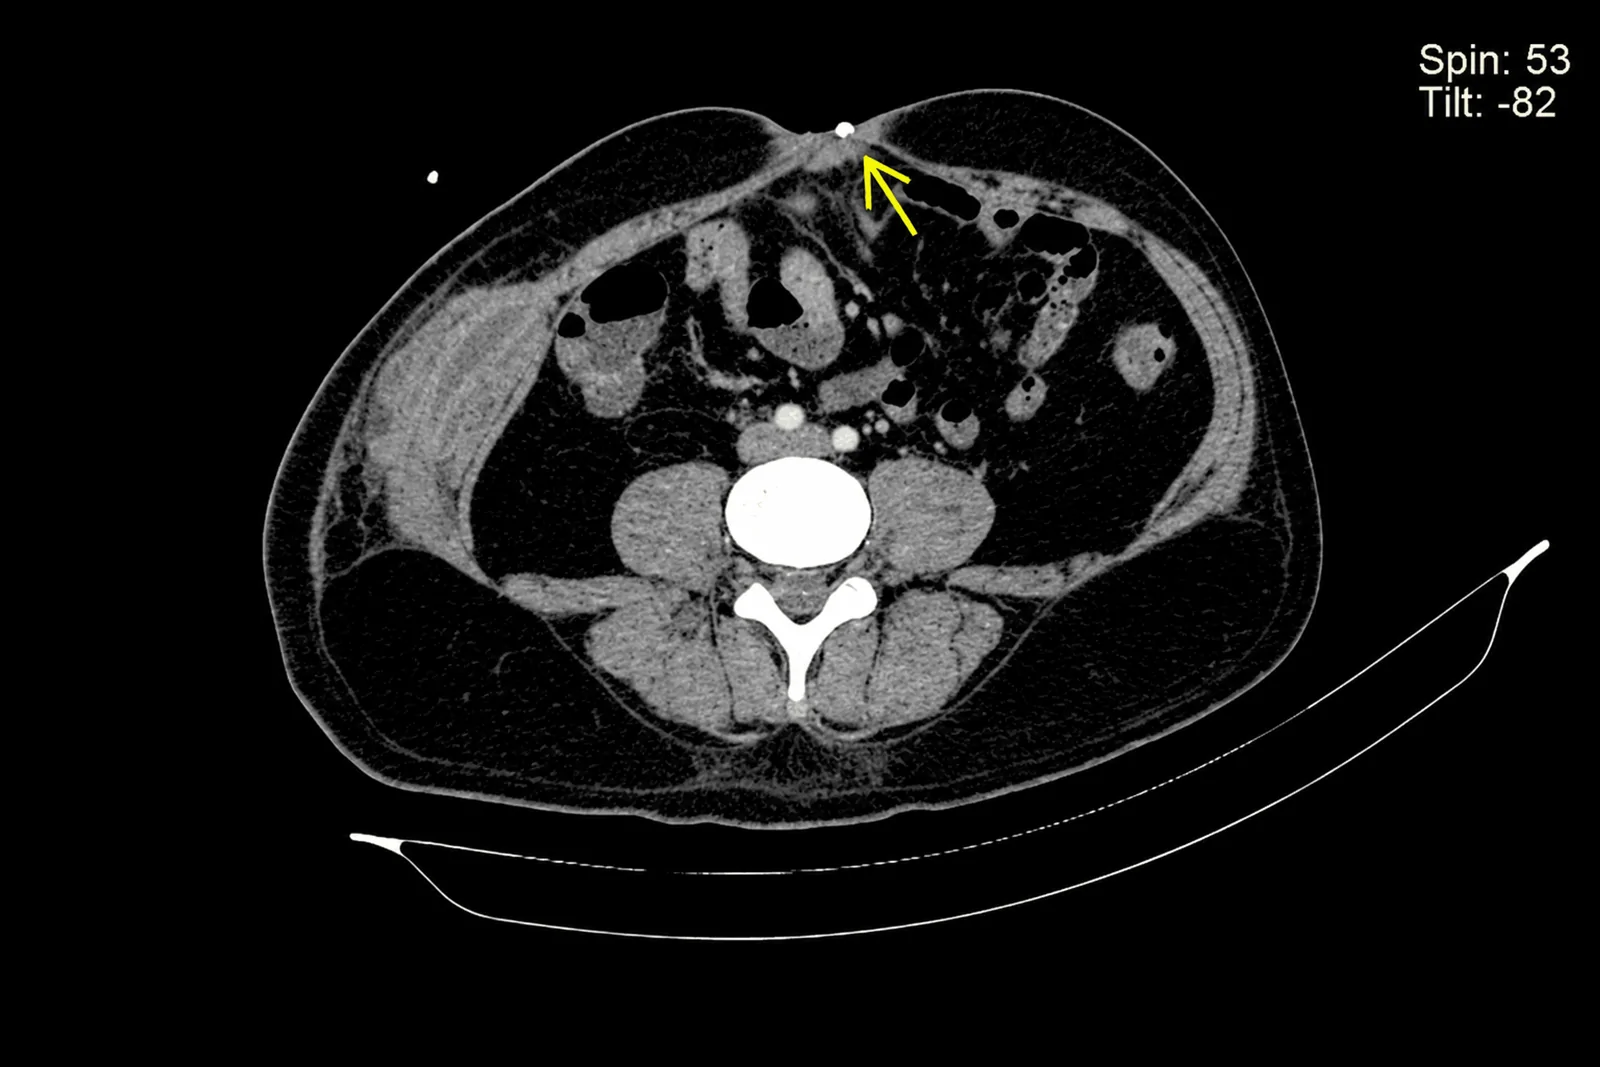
Caudal axial computed tomography image additionally showing the defect at a lower level. The arrow shows the precise location of the hernia.
